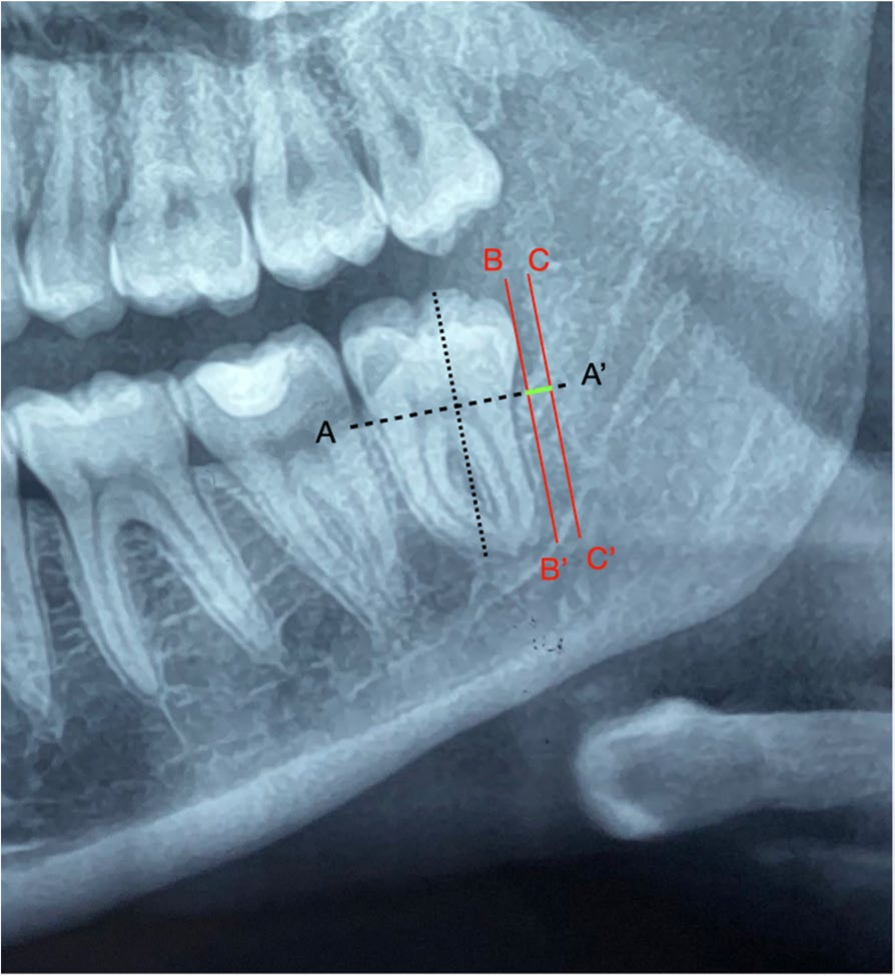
Measurement scheme on X-ray OPT for the assessment of the DALM lesion’s size
